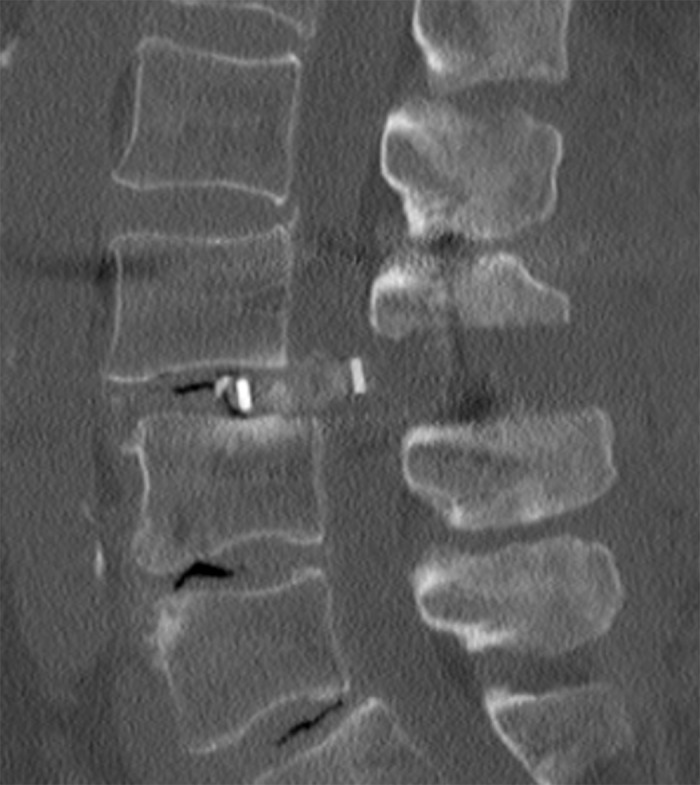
Cage Migration Computed tomography (CT) demonstrating migration and displacement of the interbody cage into the spinal canal causing severe central canal stenosis.
